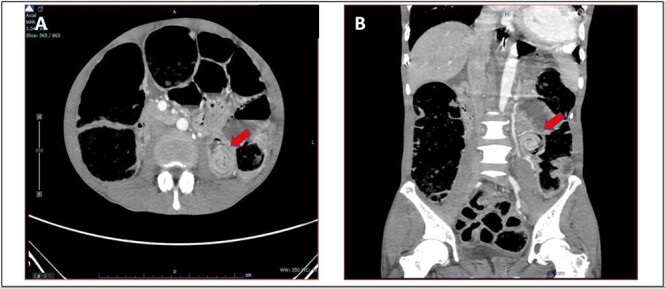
(A) Axial view and (B) coronal view of computed tomography showed presence of doughnut sign at left lumbar region suggestive of jejunojejunal intussusception (red arrow).
